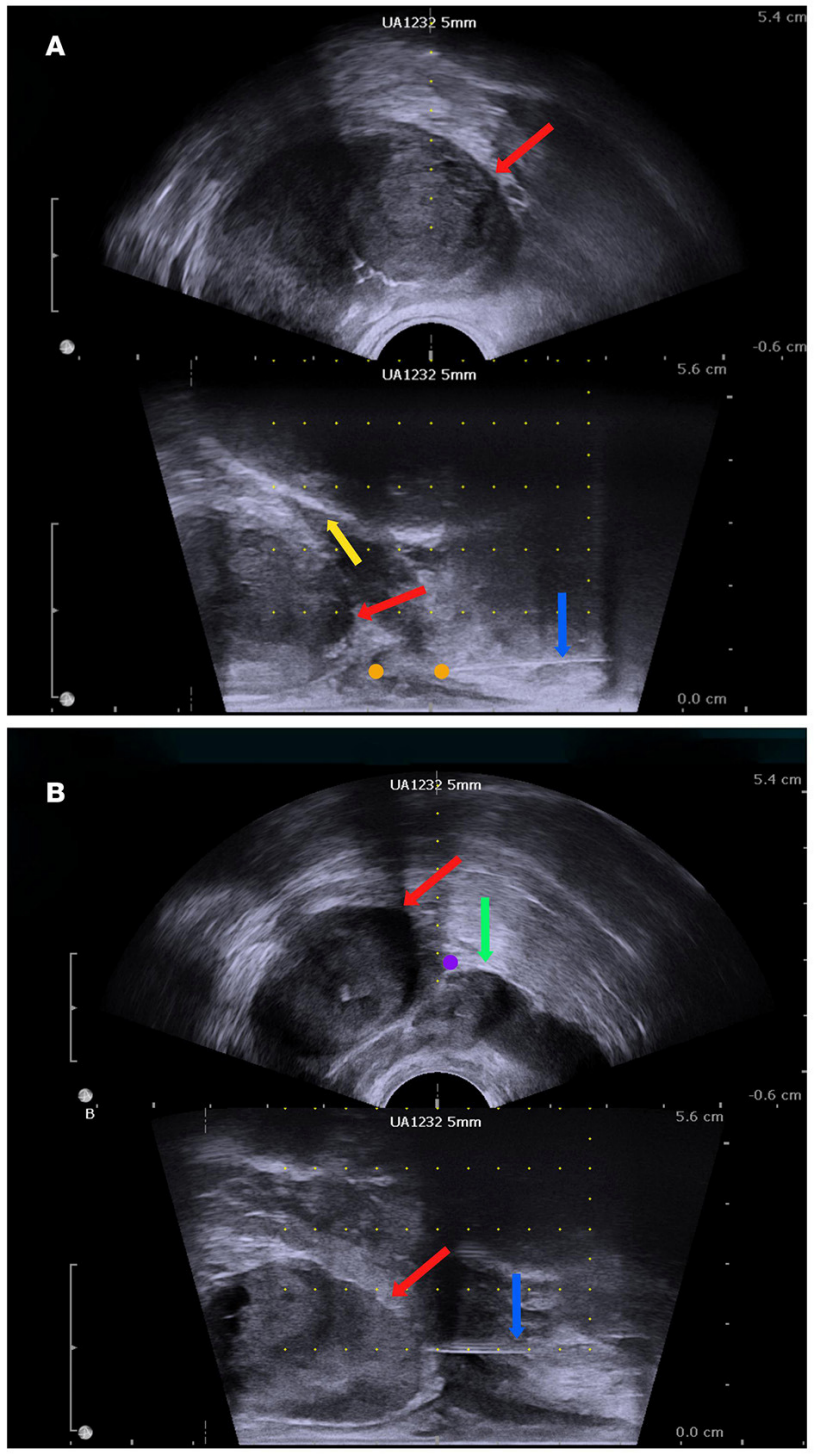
The biplanar transrectal ultrasound image. (A) Perineal nerve block. (B) Periprostatic block: prostate (red arrow); pubis (yellow arrow); seminal vesicle (green arrow); anaesthesia needle (blue arrow); perineal nerve block site (orange dot); periprostatic site (purple dot).
